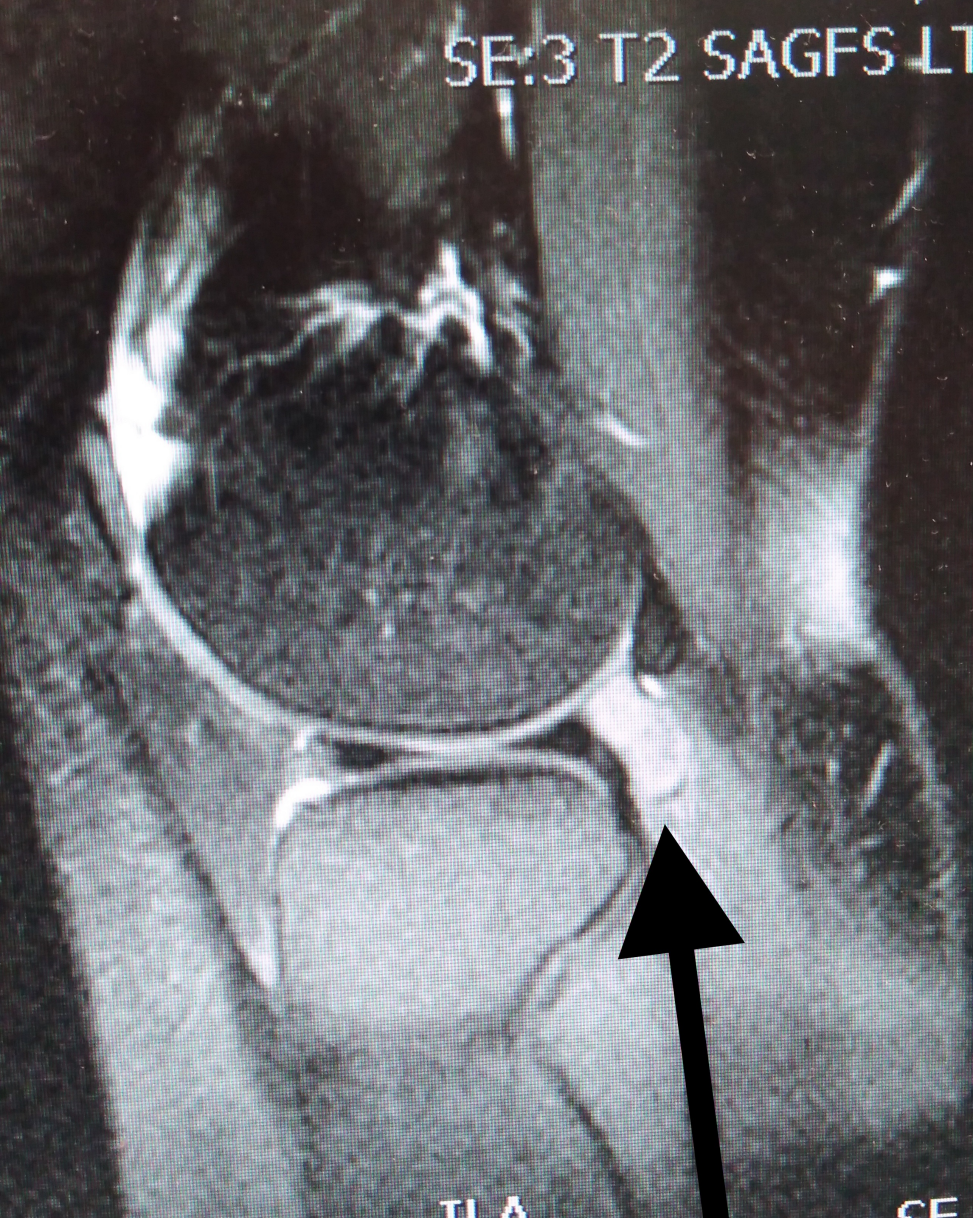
MRI of giant cell tumour MRI (T2 weighted) of giant cell tumour of popliteus tendon sheath. MRI: Magnetic resonance imaging.
